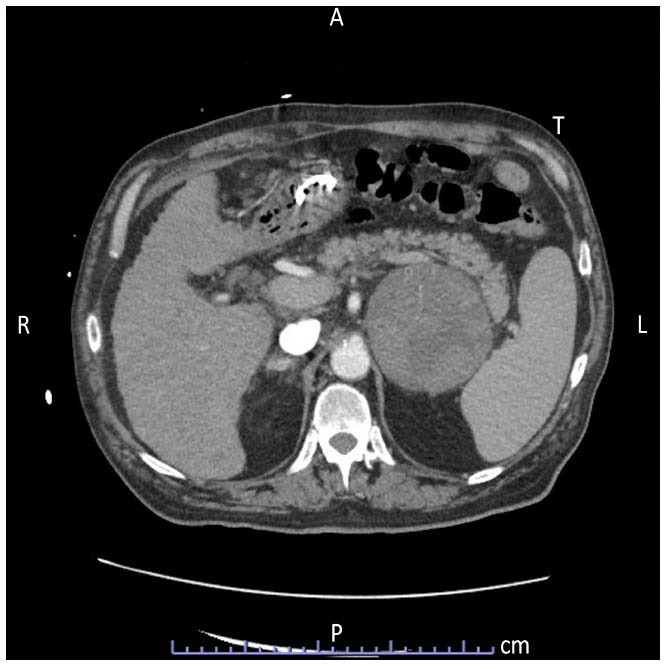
Pre-operative CT showing the left adrenal lesion.
